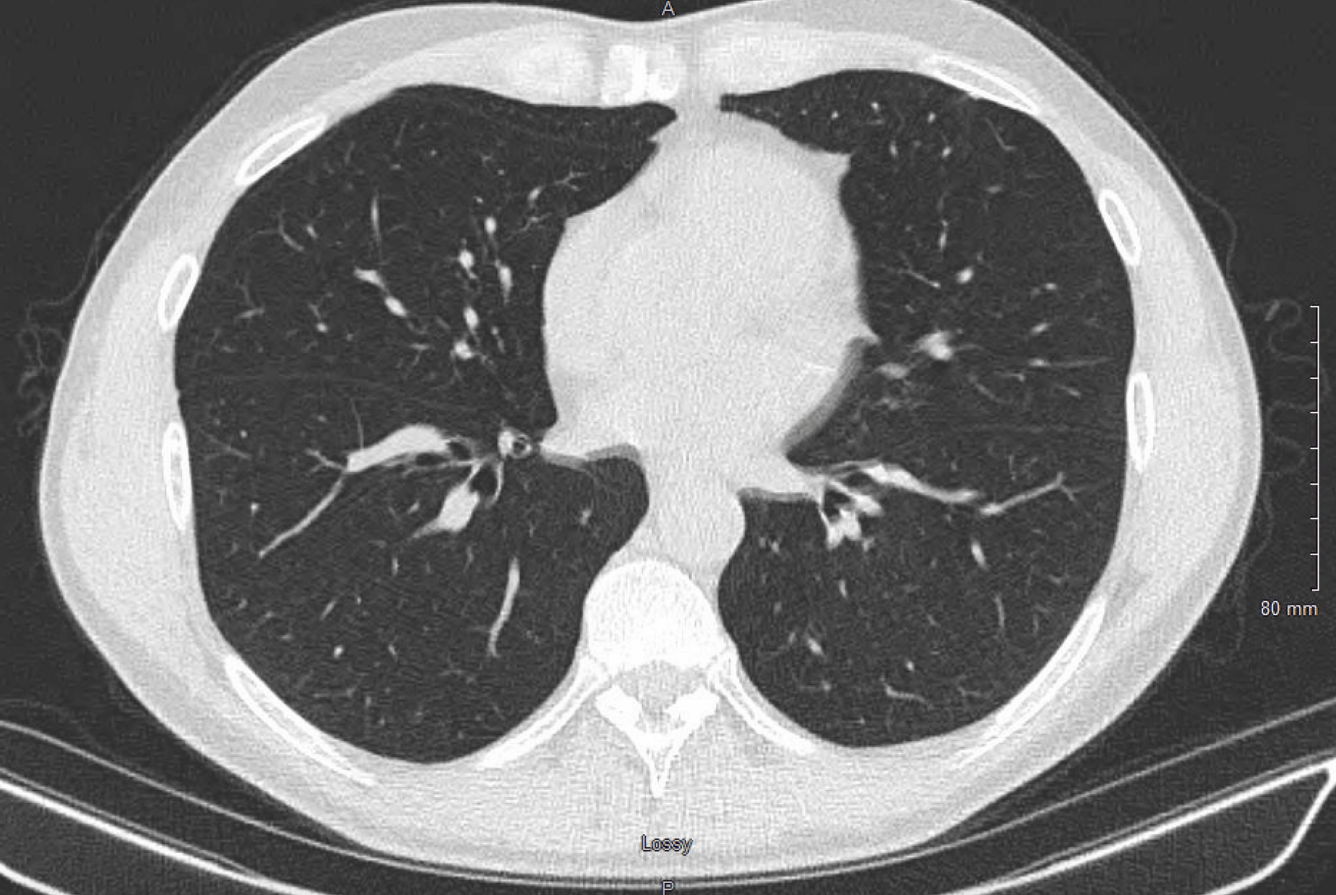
Chest CT after completion of antibiotic treatment A chest CT after the completion of antibiotic treatment demonstrates marked improvement compared to the prior study, with resolution of nodularity and ground-glass opacities in both lungs. No new nodules are identified.
